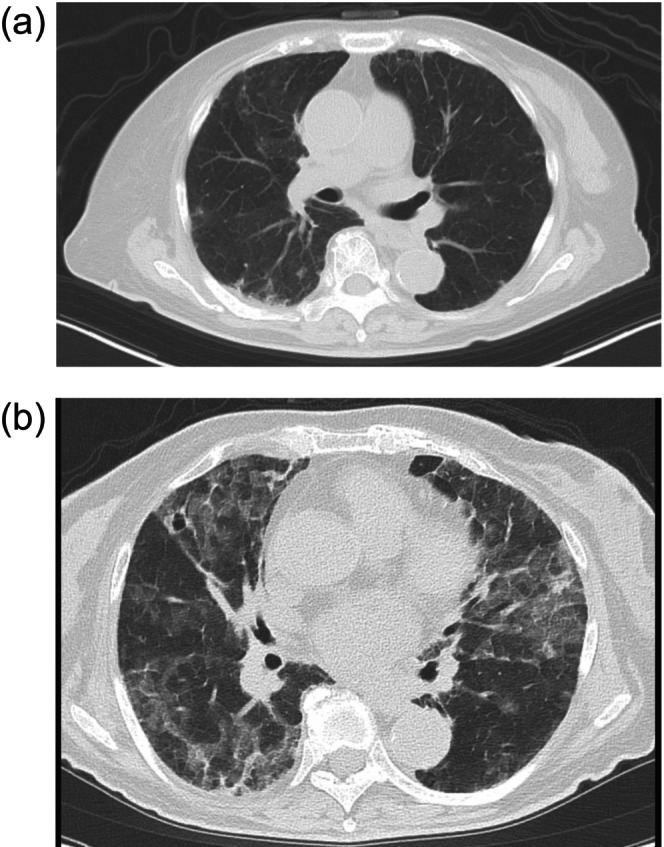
Timeline of CT scan imaging of interstitial lung disease (ILD). On day 8 of the second cycle of enfortumab vedotin plus pembrolizumab, ILD developed to grade 1. One week later, ILD worsened to grade 3. (a) Development of ILD. (b) Exacerbation of ILD. ILD, interstitial lung disease.
